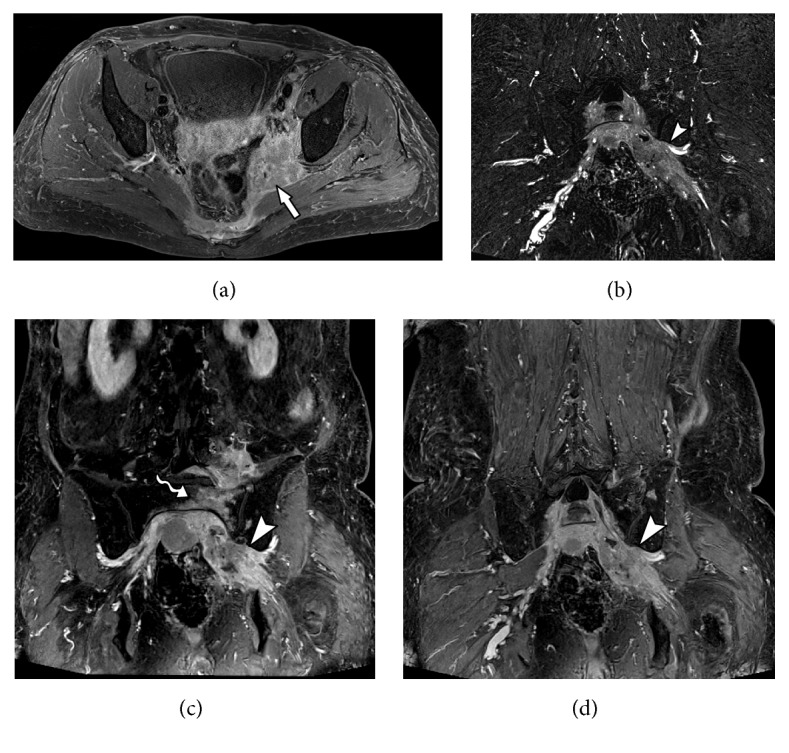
73-year-old female with pain for 4 months in sciatic territory, with history of Hodgkin lymphoma undergoing chemotherapy. MRN images in axial T1-weighted contrast-enhanced (a), coronal VIBE subtraction (b), and coronal T1 postcontrast VIBE 3D SPACE ((c) and (d)) show a large, lobular, contrasting, infiltrative mass (solid arrows) suggestive of a lymphoproliferative process, disseminated inferiorly through the left paravertebral region near the psoas and presacral region. The mass also infiltrates the left neural foramina and emergent nerve roots of L4-L5 and L5-S1 and, bilaterally, in S1-S2 to S4-S5. It determines diffuse involvement of the left sacral plexus, proximal segments of the obturator, pudendal and sciatic nerve, close to the piriformis and internal obturator muscles (arrowhead). There is also signs of bone infiltration (wavy arrow).
